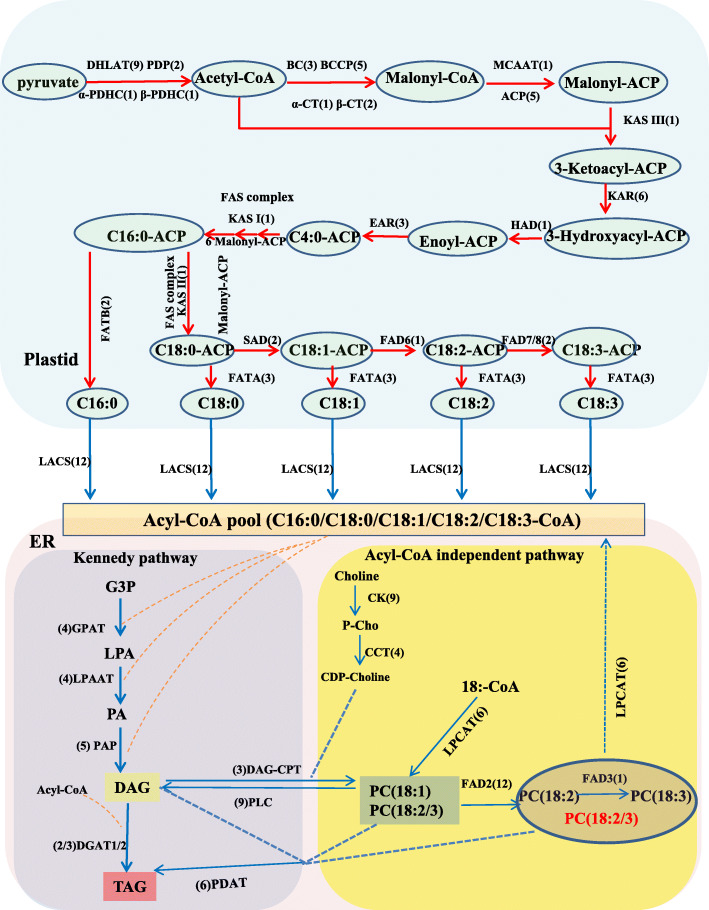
The de novo fatty acid and triacylglycerols biosynthetic pathways in safflower seeds. Note: The red and blue arrows represent the biosynthesis pathway in the plastid and ER, respectively. Lipid substrates are abbreviated: C16:0, palmitic acid; C18:0, stearic acid; C18:1, oleic acid; C18:2, linoleic acid; C18:3, linolenic acid. Enzyme/protein abbreviations are: DHLAT, dihydrolipoamide acetyltransferase; α-PDHC, pyruvate dehydrogenase alpha subunit; β-PDHC, pyruvate dehydrogenase beta subunit; PDP, pyruvate dehydrogenase phosphatase; α-CT, carboxyl transferase alpha subunit; β-CT, carboxyl transferase beta subunit; BC, biotin carboxylase; BCCP, biotin carboxyl carrier protein; MCAAT, malonyl-CoA ACP transacylase; ACP, acyl carrier protein; KAS I, II, III, ketoacyl-ACP synthase I, II, III; KAR, ketoacyl-ACP reductase; HAD, hydroxyacyl-ACP dehydrase; EAR, enoyl-ACP reductase; SAD, stearoyl-ACP desaturase; FAD6, oleate desaturase (chloroplast); FAD7/8, linoleate desaturase (chloroplast); FAD2, oleate desaturase; FAD3, linoleate desaturase; FATA/B, acyl-ACP thioesterase A/B; LACS, Long-Chain Acyl-CoA Synthetase; GPAT, glycerol-3-phosphate acyltransferase; LPAAT, 1-acylglycerol-3-phosphate acyltransferase; PAP, phosphatidic acid phosphatase; DGAT1/2, acyl-CoA: diacylglycerolacyltransferase 1/2; PLC, phospholipase C; CK, choline kinase; CCT, choline-phosphate cytidylyltransferase; LPCAT, lysophosphatidylcholine acyltransferase/lyso-PAF acetyltransferase; PDAT, phospholipid:diacylglycerolacyltransferase; DAG-CPT, diacylglycerol cholinephosphotransferase
